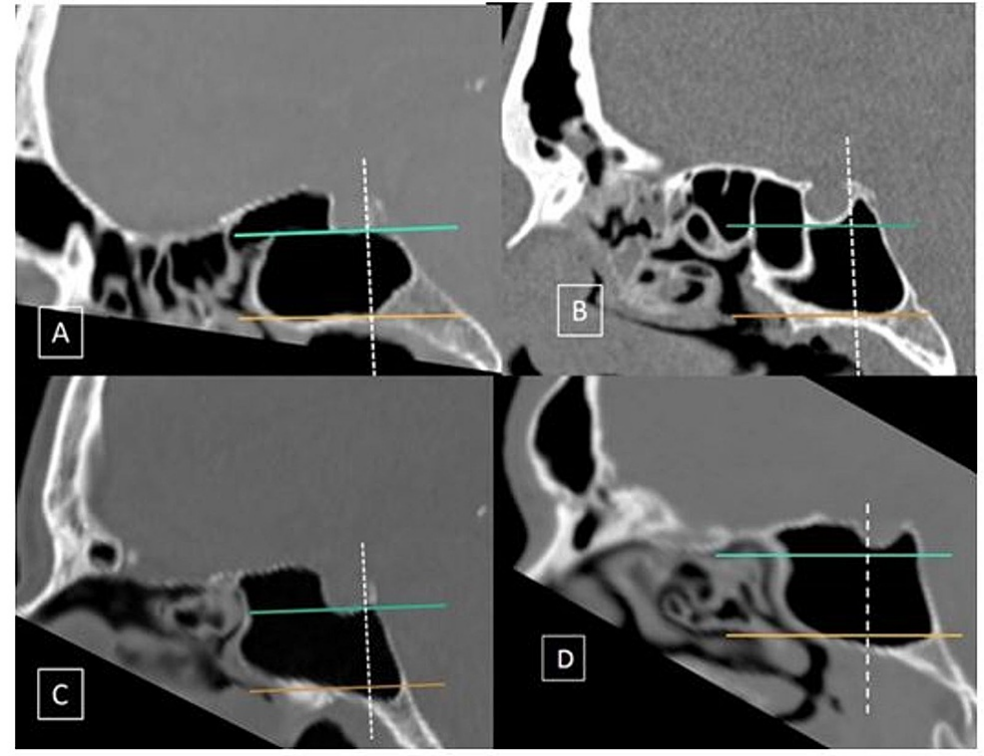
Classification of craniocaudal extension of sellar pneumatization in the sphenoid sinus CT bone window mid-sagittal sections showing the various types of sphenoid pneumatization. Horizontal lines are drawn along the inferior margin of the sella (green line) and the vidian canal (orange line). The vertical line is along the posterior wall of the sella (dotted white line). A: Pneumatization does not extend above the inferior margin of the sella or below the level of the vidian canal. B: Dorsal; pneumatization extending into the dorsum sella. C: Occipital; pneumatization that extends inferiorly to the vidian canal level. D: Combined; dorsal + occipital CT: computed tomography
